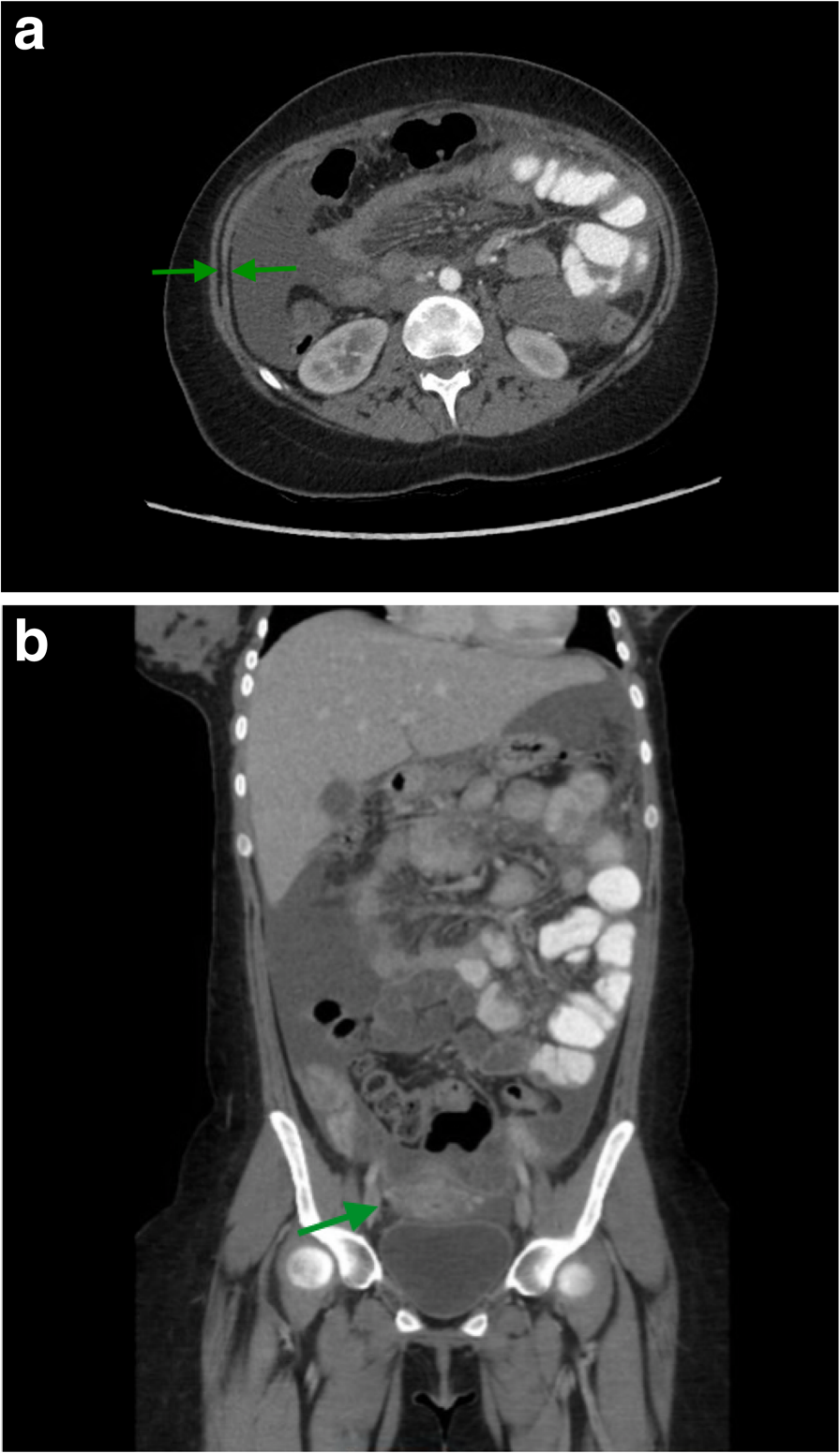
a and b. Contrast-enhanced CT images of the abdomen showing peritoneal thickening and hypervascular adnexes with a small para-uterine abcedation on the right side
